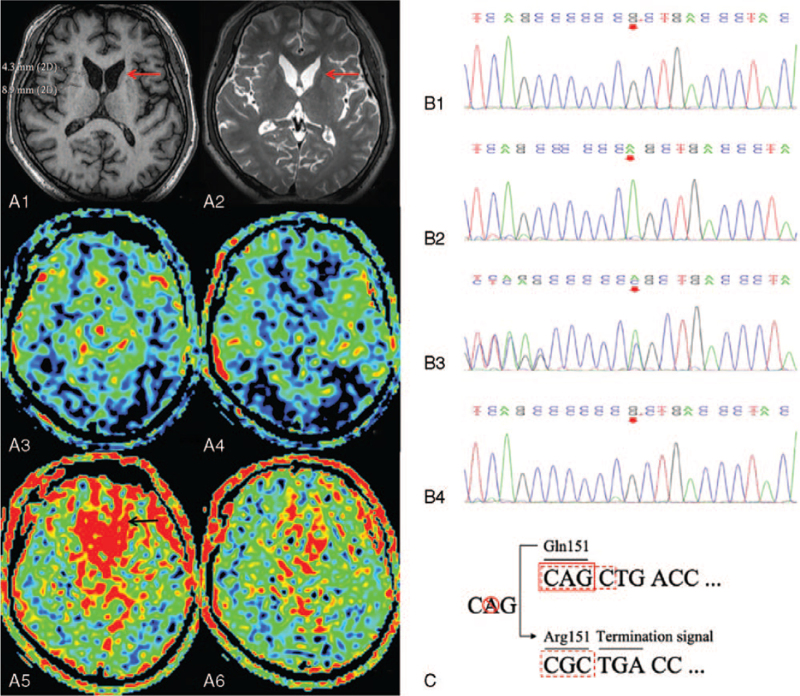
(A) The brain MRI of the patient with McLeod syndrome. (A1–A2) Axial view of T1 weighed image (A1) and T2 weighed image (A2) of the patient showed significant atrophy of bilateral caudate nuclei (red arrows) and mild atrophy of bilateral putamen. (A3–A6) Arterial spin labeling (ASL) images revealed a reduction in total cerebral perfusion (A3–A4) and an increase of the delayed frontobasal perfusion (A5–A6, see black arrow in A5). (B) Sequence analysis of the patient with McLeod syndrome and his family. (B1) Proband: a frameshift mutation in the *XK* gene. (B2) Father: no mutation in the *XK* gene. (B3) Mother: heterozygote of the *XK* gene. (B4) Brother: a frameshift mutation in the *XK* gene. (C) The frameshift mutation resulted in an amino acid sequence change from Gln to Arg-termination signal. MRI = magnetic resonance imaging.
